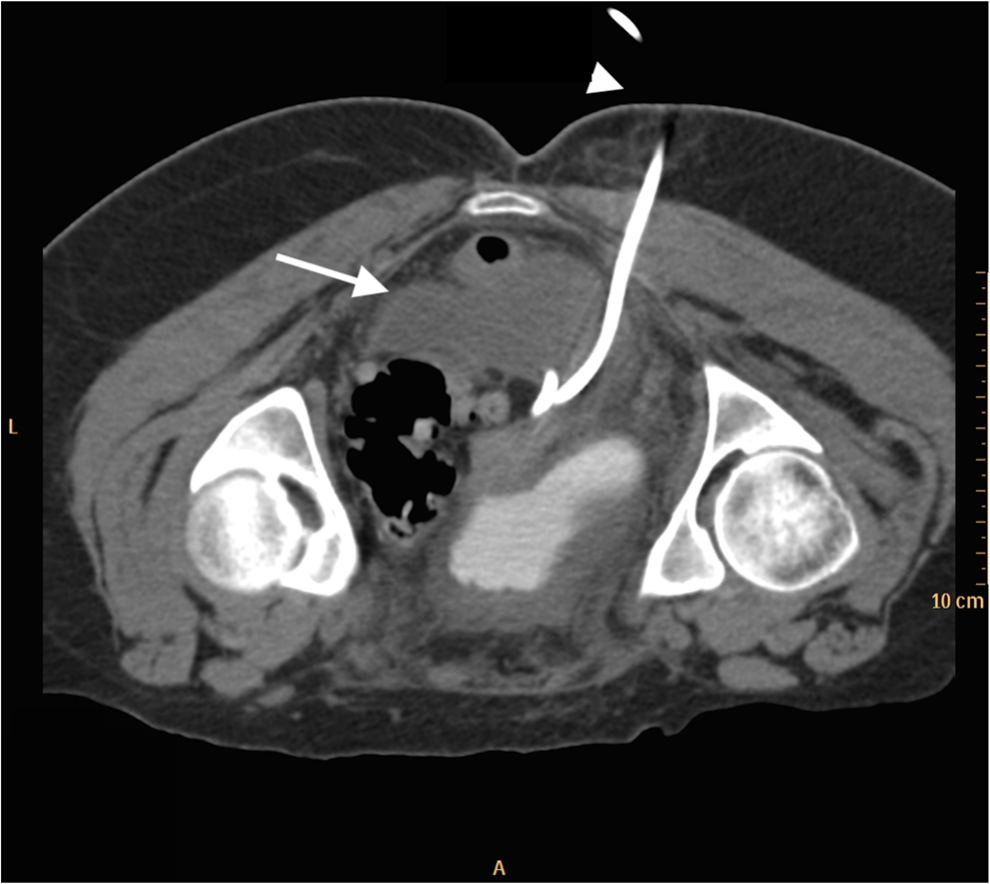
From patient #3. Transverse delayed post-contrast CT of the pelvis. The patient is in a prone position. There has been interval placement of a right-sided transgluteal drainage catheter (*arrowhead*) in to the posterior pelvic abscess (*arrow*), which was also seen in Fig. 1
